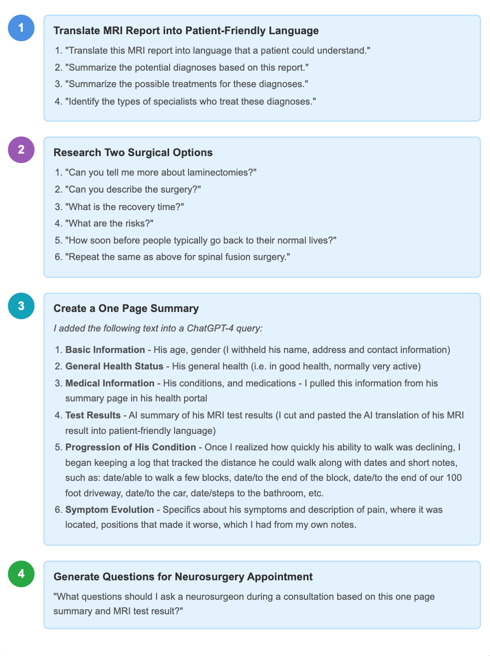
Approach used with generative artificial intelligence by the family caregiver in the case presented. MRI: magnetic resonance imaging; AI: artificial intelligence.
